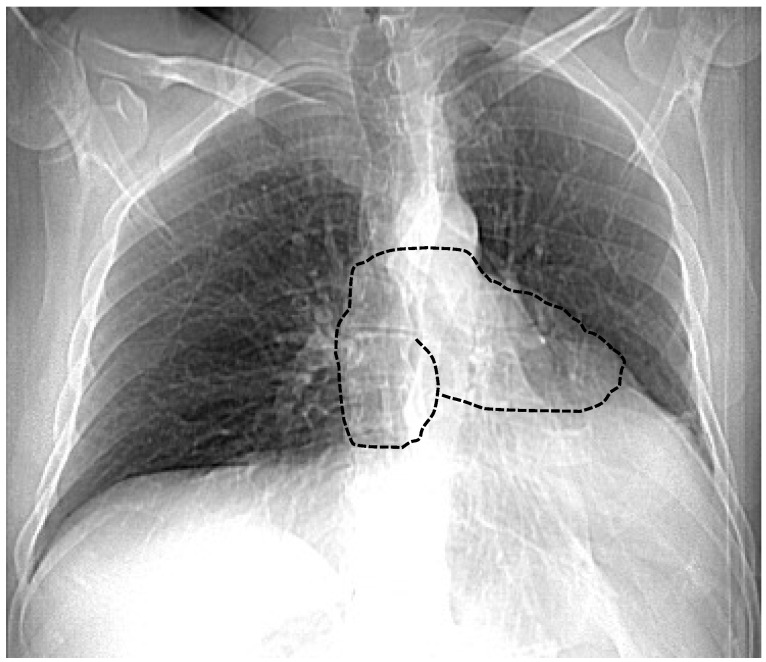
Chest X-ray of a patient with CAP. The right heart border is obscured by vertebral spine while the left edge results in fattening and elongation; this is called the “Snoopy signs” (drawn in black line).
